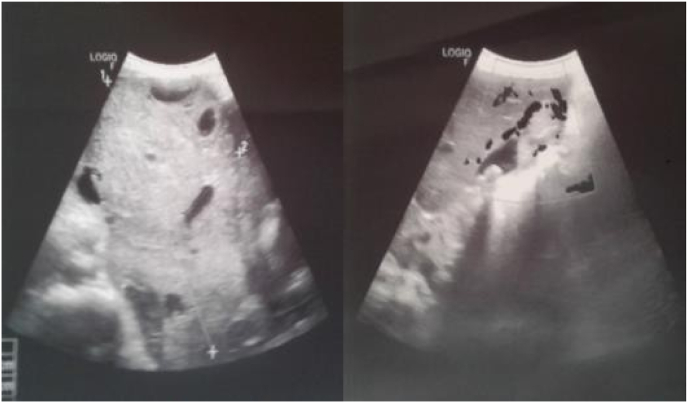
Abdominopelvic ultrasound scan showing a large abdominopelvic mass, reaching the uterus, oblong, echogenic, heterogeneous, vascularized on Doppler, with multiple poorly defined cystic formations, measuring 18x10 × 11 cm.
